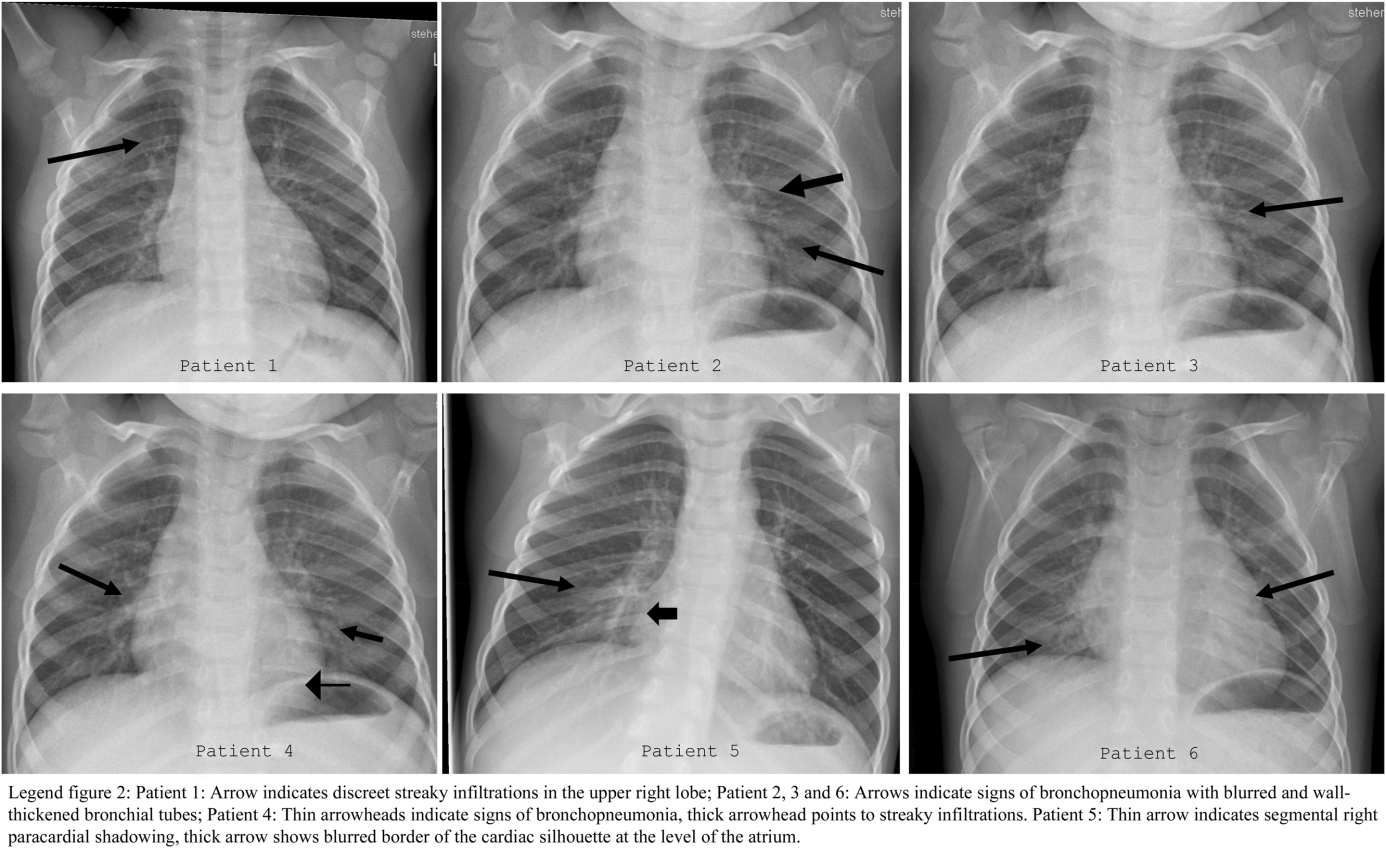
Chest X-rays of six children with pulmonary tuberculosis prior to therapy
